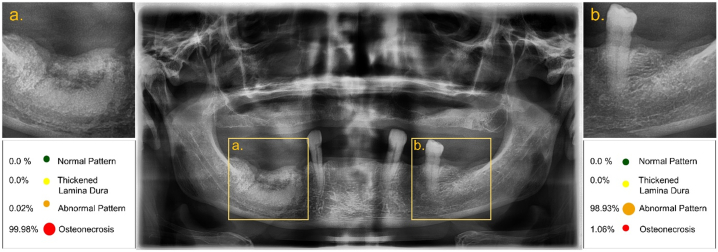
Display of the interface using a panoramic radiograph of a 70-year-old patient presenting with clinical bone exposure in the right posterior mandible. No other lesions were observed on the clinical examination. Once the image is imported, manual selection of different regions of interest (a, b) can be performed. The output will show the category to which the selected trabeculated region belongs together with the corresponding probability in the form of a colour-coded index.
